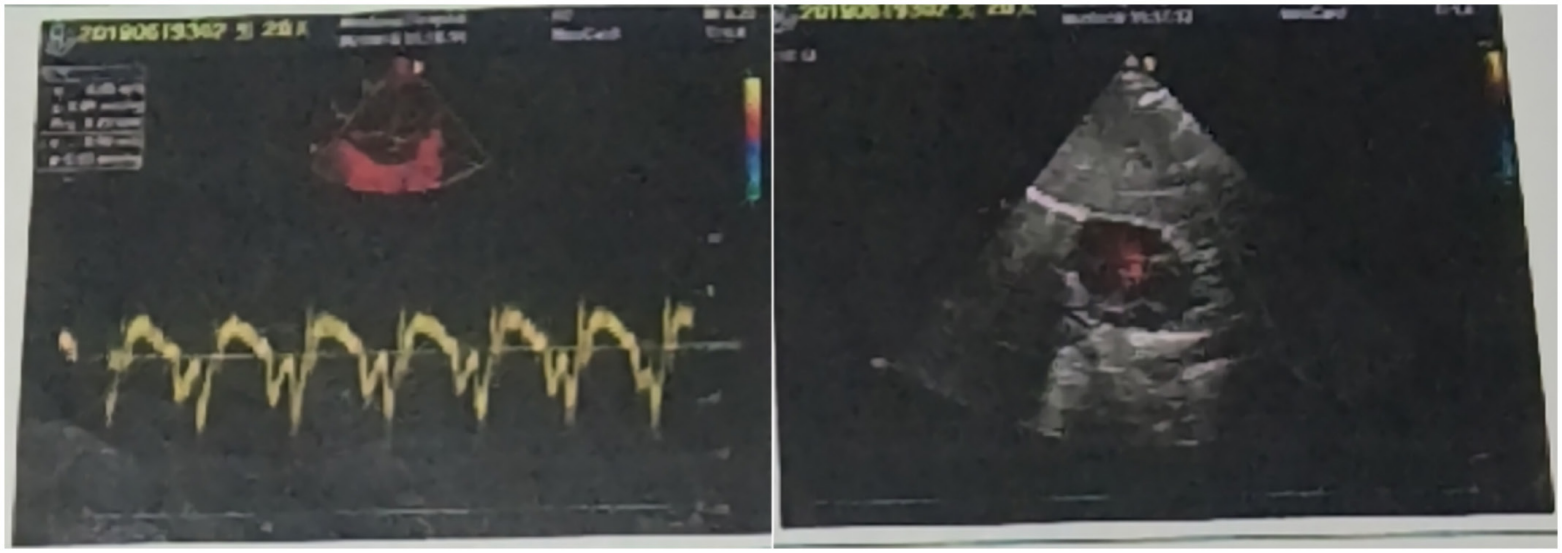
Doppler ultrasound showed multiple heart defects in the patient
